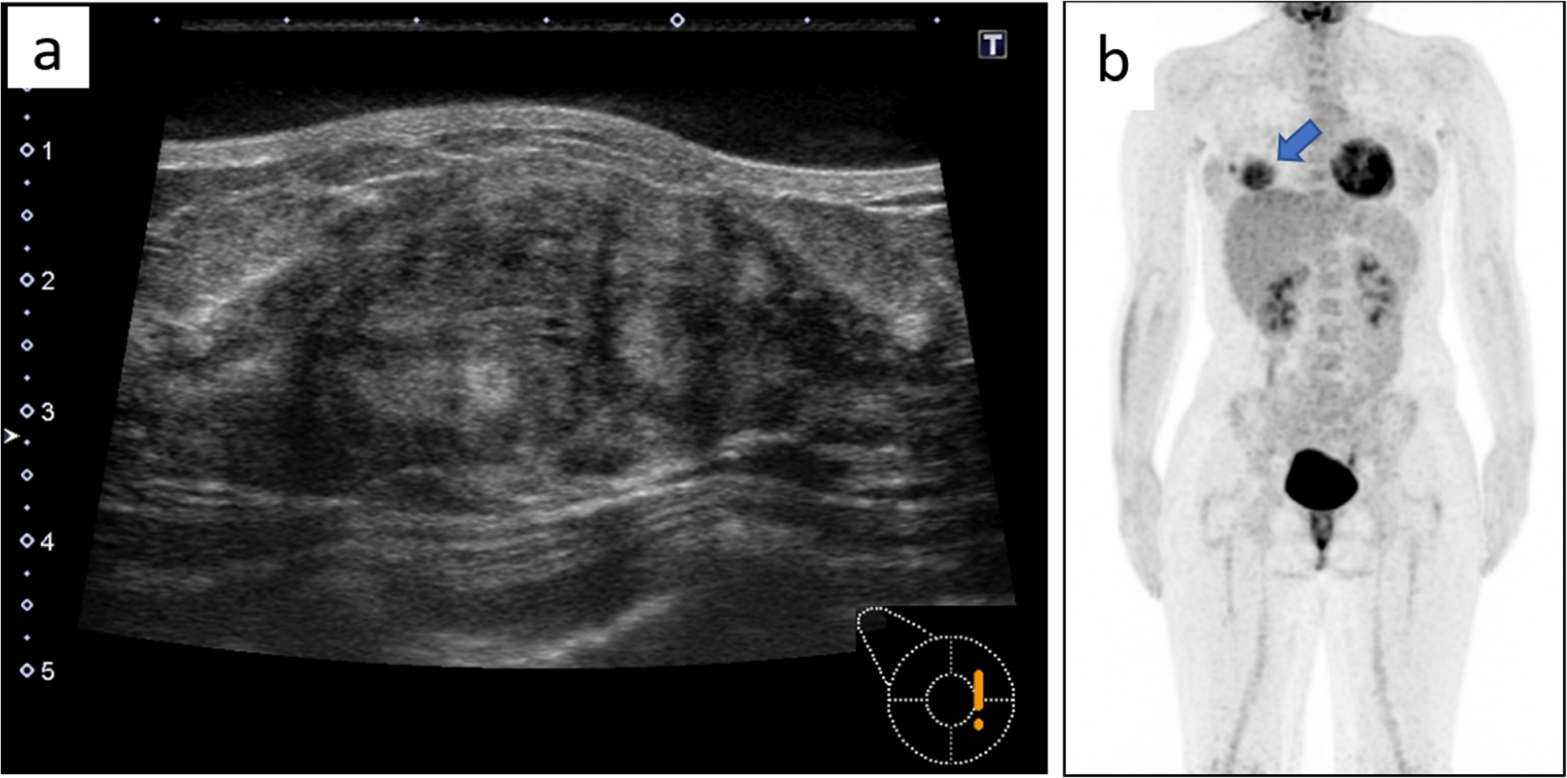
Findings of ultrasound and PET-CT scan. a Ultrasonography revealed a 40-mm tumor with an irregular shape. Internal echo was a mixture of low and high levels, suggesting that fibroadenoma, breast cancer, and sarcoma should be included in the differential diagnosis, along with lymphoma. b PET-CT scan showed high FDG uptake in the right breast (blue arrow). There were no abnormal hot spots in other organs, including the ipsilateral axilla
